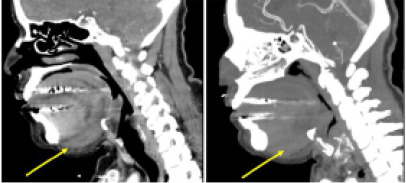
Computed tomography imaging with contrast (on the left) and angiogram (on the right) demonstrates soft-tissue swelling (yellow arrows) in the hypopharynx without any active bleeding. Images are slightly distorted due to dental artifacts
